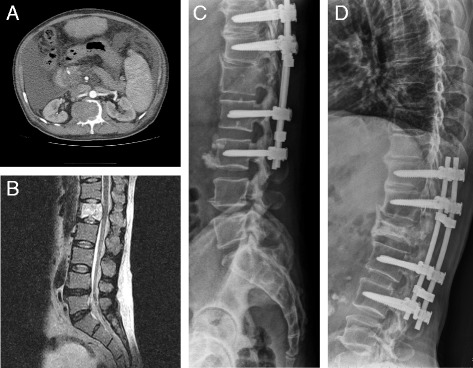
Preoperative and postoperative images of a 49-year-old man with L1 vertebral metastatic spinal cord compression from pancreatic cancer who underwent surgery using the posterolateral transpedicular approach without anterior vertebral reconstruction. The CT angiography (a) and MRI image (b) demonstrate metastatic spinal tumor with cord compression at L1. The immediate postoperative radiographs (c) and the 28-month follow-up radiographs (d) demonstrate no screw loosening or implant failure
